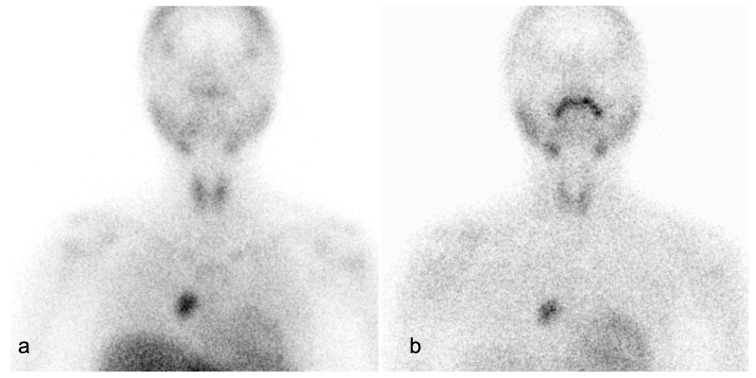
A 23-year-old female with pain in bilateral hip, knee and elbow joints associated with weakness in walking. Ultrasonography (USG) was normal. Computed tomography (CT) showed a 6.8 × 10.7 × 15.4 cm enhancing lesion in suprasternal space posteriorly, abutting the left infrahyoid strap muscle between the brachiocephalic trunk and left common carotid artery (CCA), suspicious for ectopic parathyroid adenoma in suprasternal space. (a) Early static views show areas of increased tracer uptake in both lobes of the thyroid, with focus of abnormal tracer concentration in the superior mediastinum at the right paracardiac region. (b) Delayed image at 3 h post-injection shows almost complete washout of the tracer from both lobes of the thyroid, with persistent tracer uptake at the right paracardiac region.
